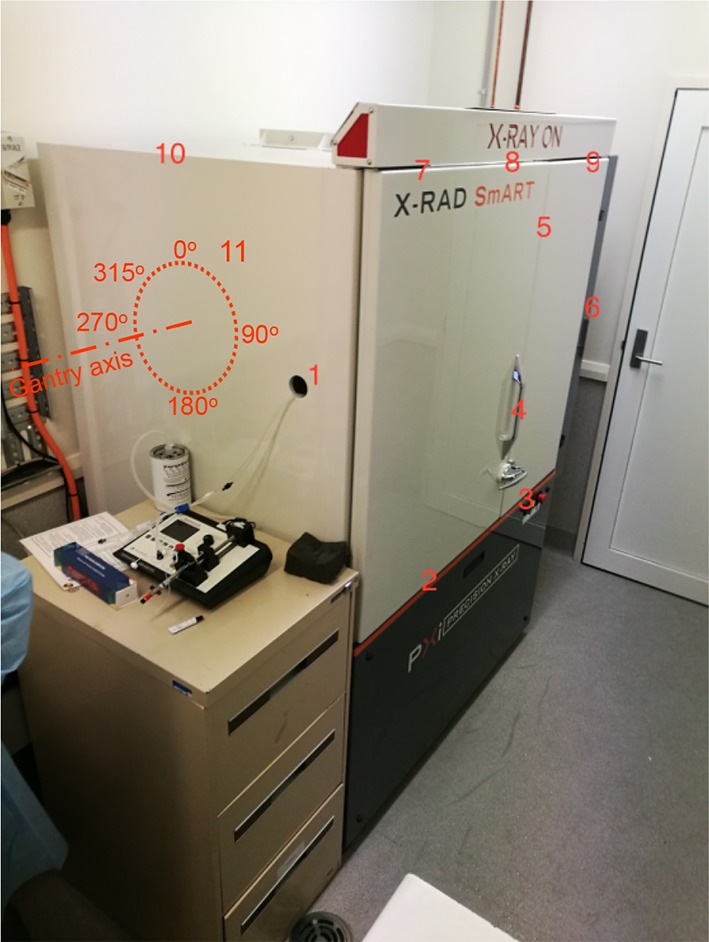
The location of the 11 survey points investigated during radiation safety survey. Point 11 remained aligned with the X-ray tube cathode–anode axis (gantry angle indicated)
